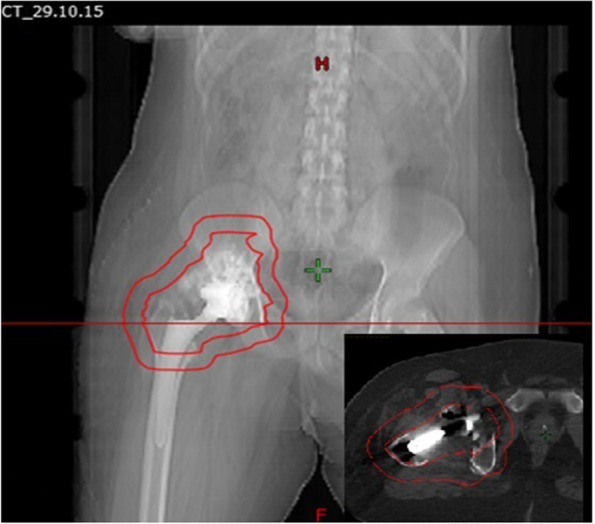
Hip heterotopic ossification contouring. Orthogonal view of contouring a patient with Rt hip heterotopic ossification performed total hip arthroplasty in addition to CT slice showing clinical target volume and planning target volume
